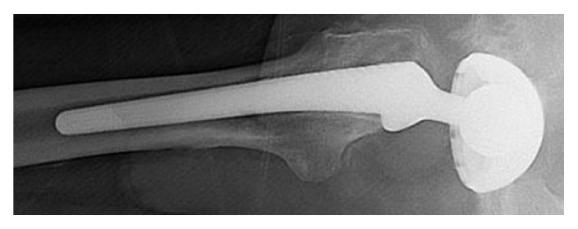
Lateral X-ray shows medial migration of the femoral head within the acetabular shell and excludes a dislocation as it confirms the prosthetic femoral head lies within the acetabular shell on a tangential view.
